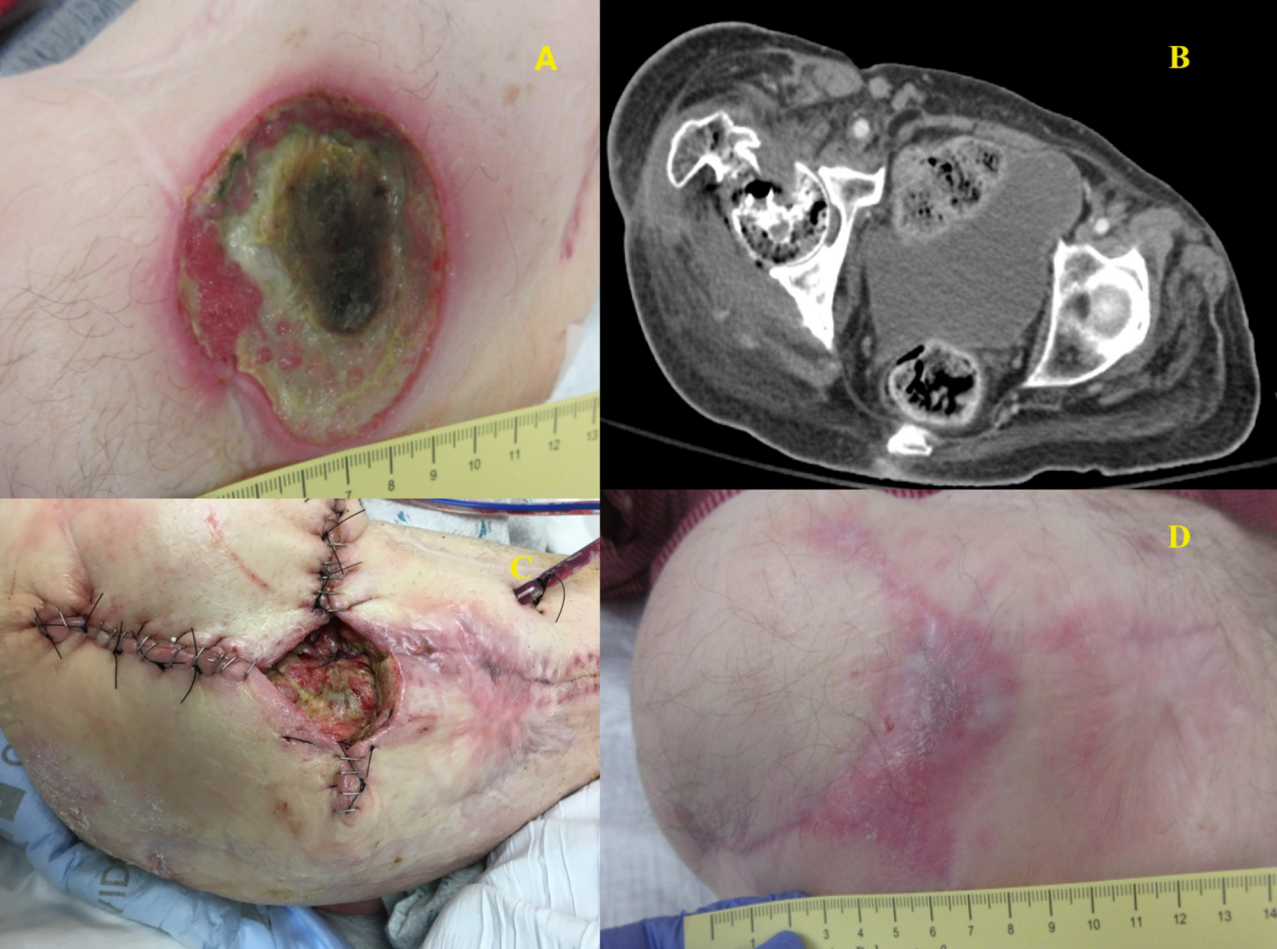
Patient 3 A: Greater trochanter ulcer; B: Pre-operative CT scan; C: Partial closure; D: Healed wound
